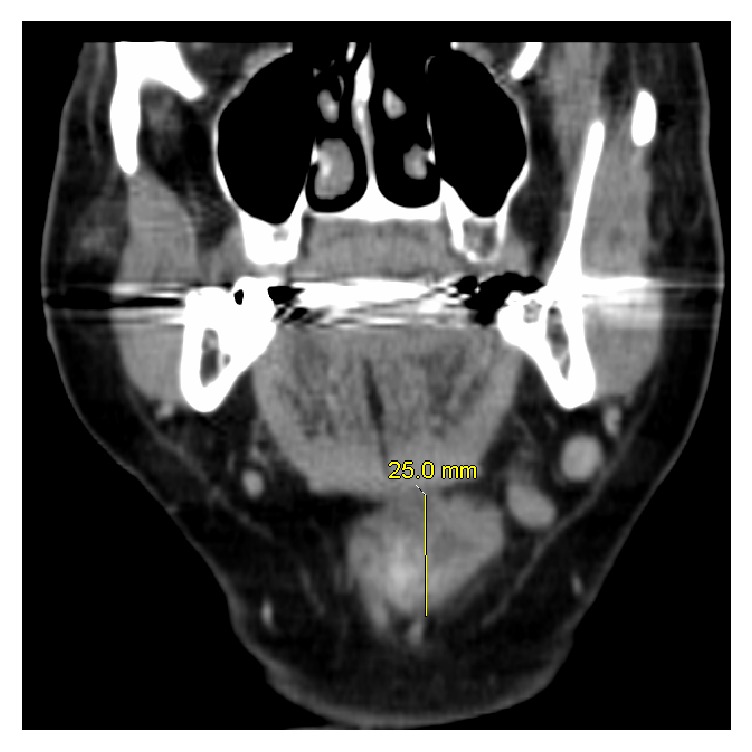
Axial view of the CT neck that showed presence of a neck mass measuring 25 mm in superior to inferior dimension.
